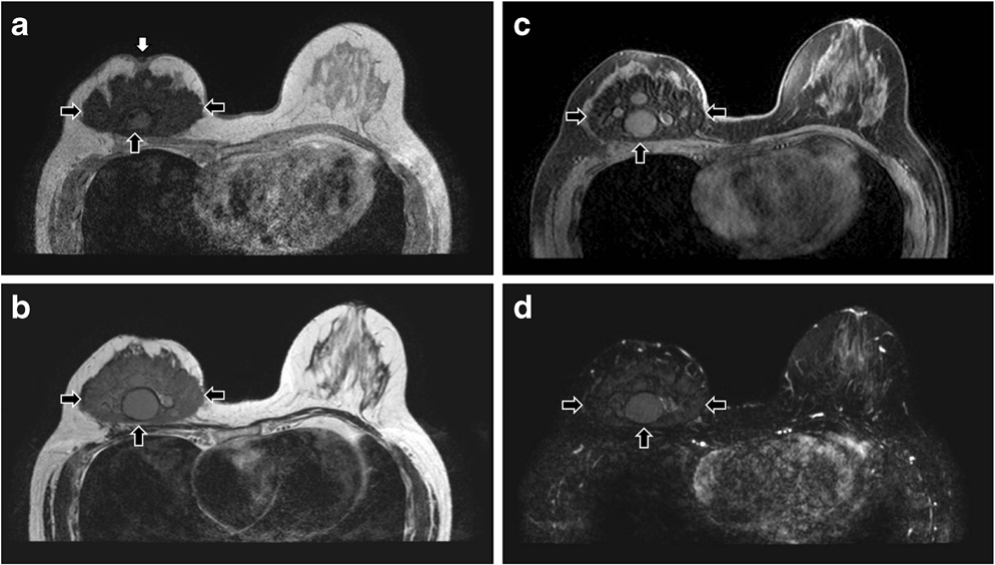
Unilateral right breast paraffin injection. T1-weighted (a), T2-weighted (b), T2-weighted, fat-saturated (c), and T1-weighted, fat-saturated, post-contrast (d) axial MR images show a right breast mass (black arrows) with T1 and T2 low to intermediate signals and minimal contrast enhancement. Nipple retraction (white arrow) is observed (a)
